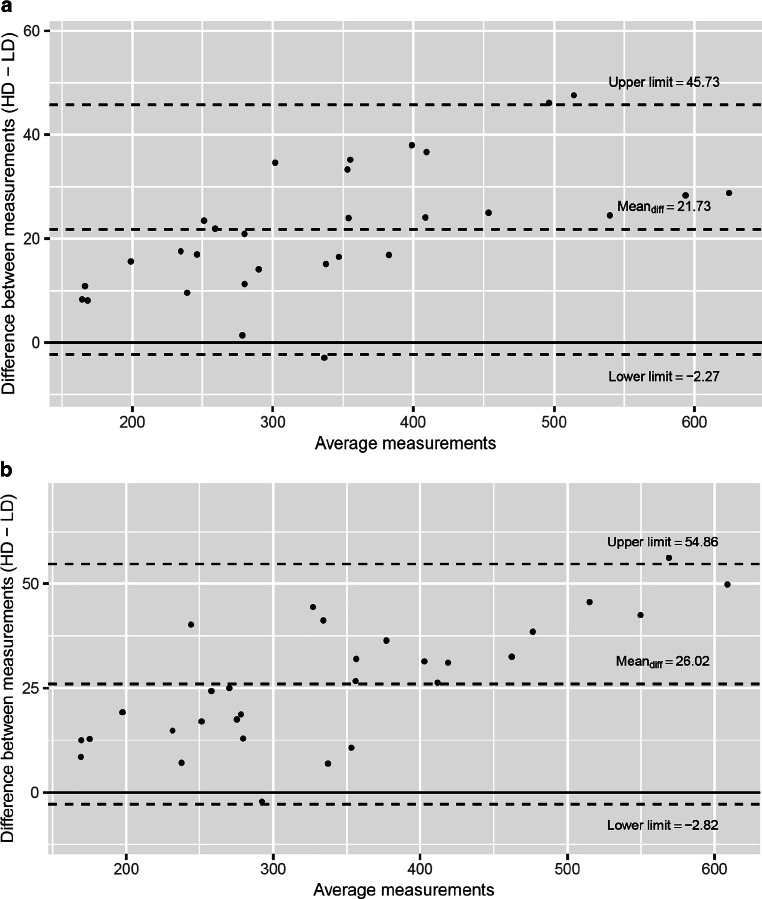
Bland–Altman plots of low-dose (LD-CBCT) and high-dose cone-beam computed tomography (HD-CBCT) volume measurements (mm^3^) from a the first round of measurements and b the second round of measurements Bland-Altman-Plots der Zahnvolumenmessungen (mm^3^) mit der dosisreduzierten (LD-CBCT) und der high-dose digitalen Volumentomographie (HD-CBCT) a aus der ersten und b aus der zweiten Messrunde
